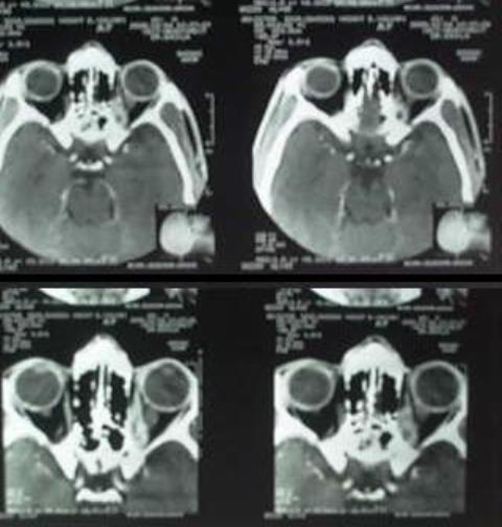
CT orbits showing multiple small non enhancing well defined hypo dense lesion in medial rectus and superior rectus muscles of left orbit.
